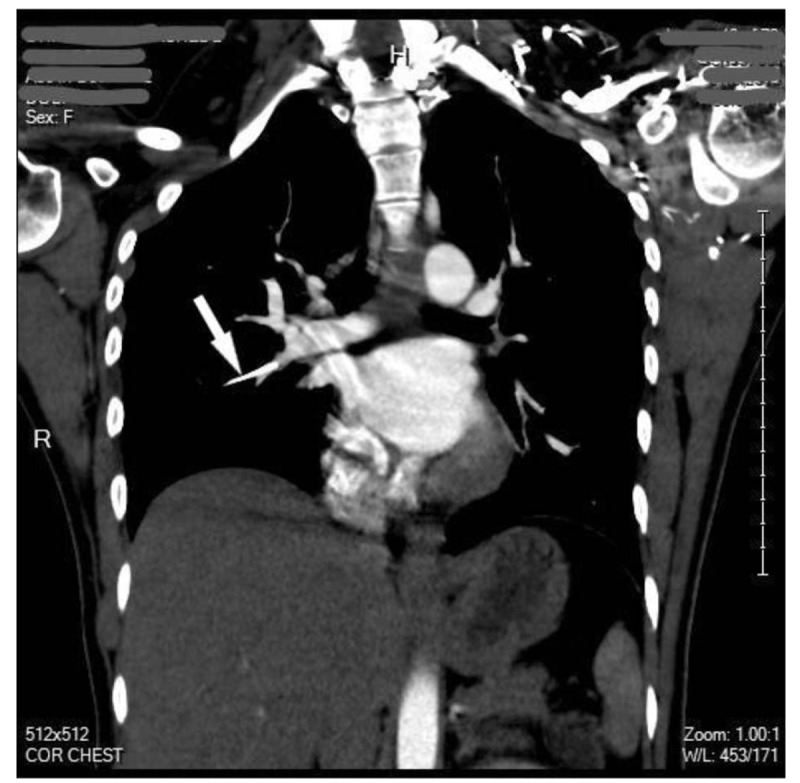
CT chest angiography showing the migrated Nexplanon (arrow) in the right middle lobe pulmonary artery.
